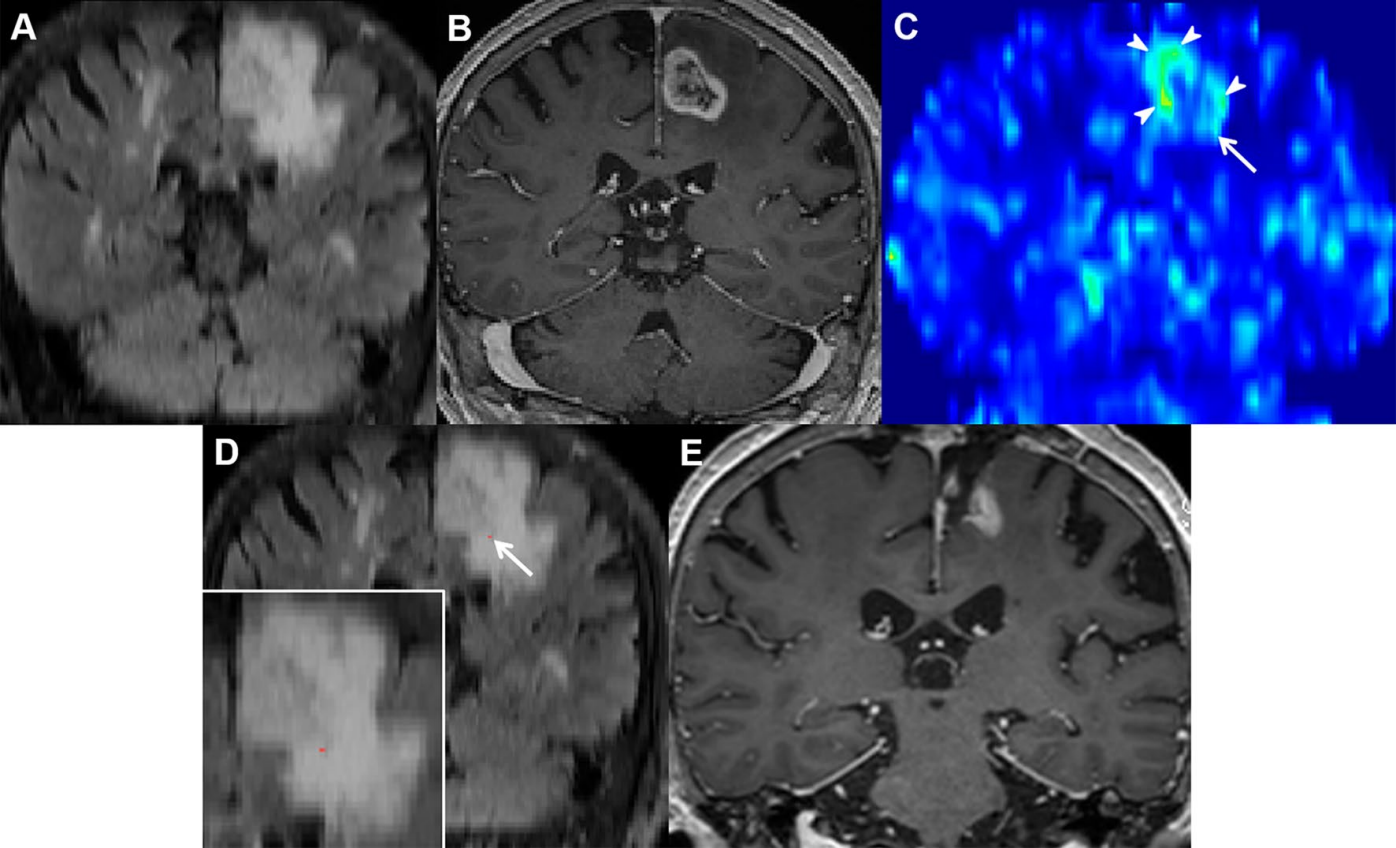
A 71-year-old woman with glioblastoma in the test set. (A,B) On coronal preoperative T2 FLAIR and CE T1W images, a heterogeneously enhancing mass was noted in the left parietal lobe along with extensive perilesional T2 hyperintensity. (C) The nCBV map depicts a focal mild increase at the inferior aspect of the enhancing tumor (arrow) along with a definite increase at the enhancing portion of the tumor (arrowheads). (D) The multiparametric MRI model predicted early local progression to occur at the red area inferior to the enhancing tumor (magnified view at the lower right corner). (E) Follow-up MR images at 7 months demonstrated a measurable enhancing lesion at the inferior aspect of the surgical cavity. *FLAIR* fluid-attenuated inversion recovery, *CE T1W* contrast-enhanced T1-weighted.
